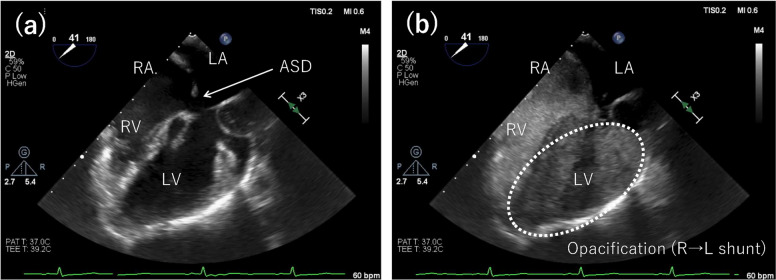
Agitated saline contrast (ASC) transesophageal echocardiography in the mid esophageal four chamber view during the modified Valsalva maneuver under general anesthesia. a Before ASC injection. b During the ASC study under general anesthesia with a modified Valsalva maneuver, the entire left ventricle was filled with contrast. RV, right ventricle; RA, right atrium; LV, left ventricle; LA, left atrium; R → L, right to left; ASD, atrial septal defect
